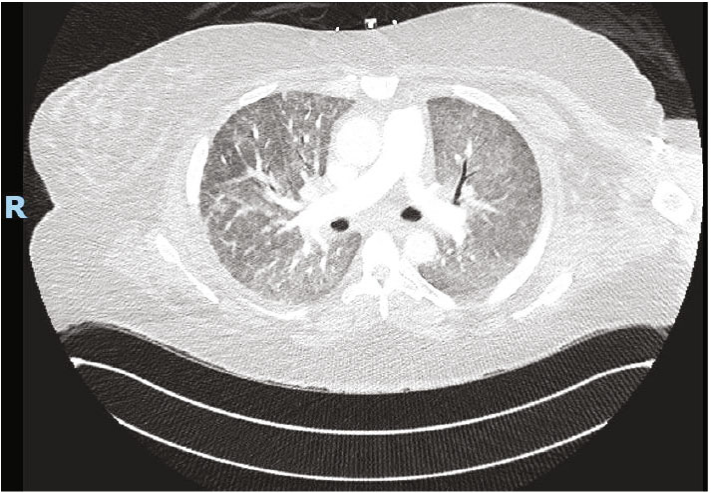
Computed tomography of the chest with angiography. Diffuse ground glass and reticular opacities are present bilaterally with areas of subpleural sparing. There is also mild bronchiectasis. There is no air trapping, honeycombing, or head cheese sign.
